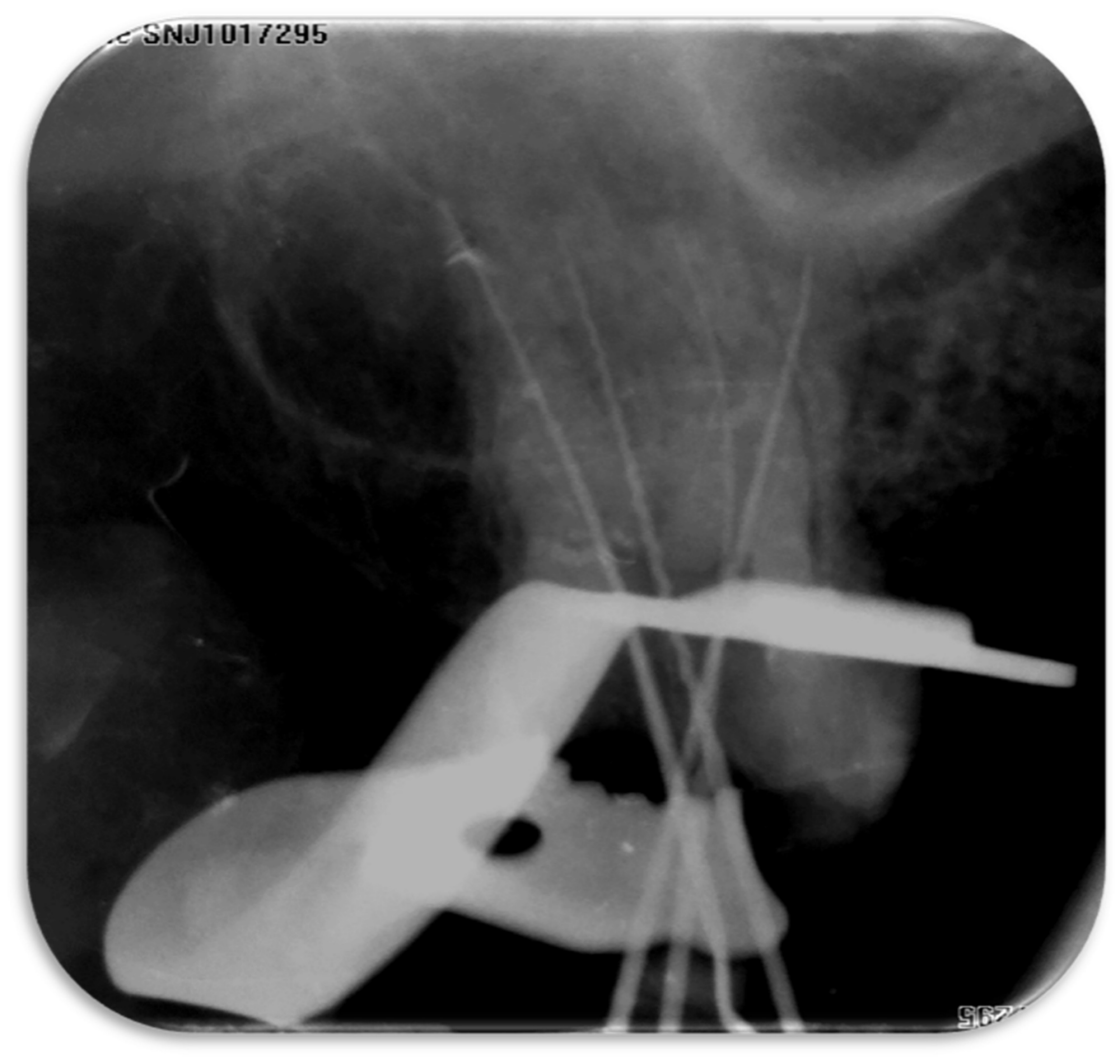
Radiographic working length determination (#27)
